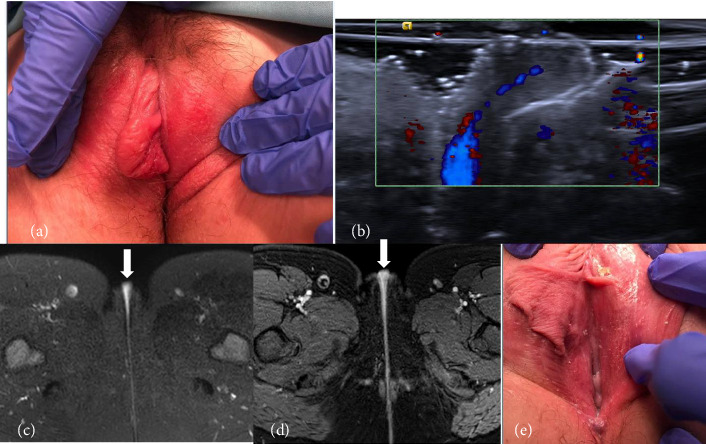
Patient 1 initial examination, imaging (ultrasound and MRI), and intraoperative findings. (a) Outpatient examination revealing an enlarged right labium minus “mass.” (b) Transverse high-resolution ultrasound with color Doppler confirms a hypoechoic solid lesion. (c) Axial T2-weighted MRI demonstrates a hyperintense lesion (arrow). (d) Postcontrast axial T1-weighted images with fat saturation show enhancing labial mass (arrows). (e) Intraoperative findings of an elongated right labium minus.
